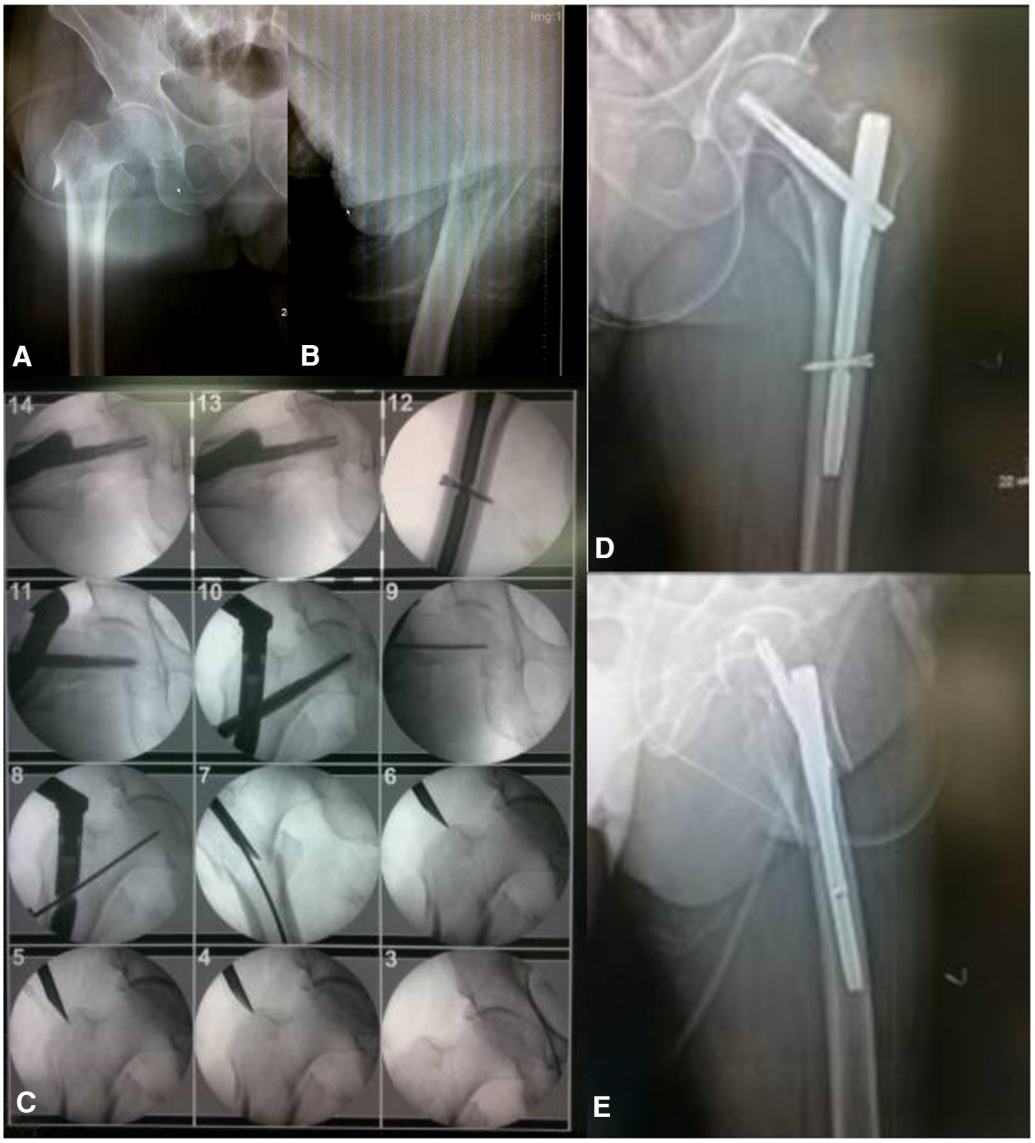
Preoperative anterolateral x-rays of the patient's hip joint (A,B) surgical procedure for PFNA (C) postoperative anterolateral x-rays of the patient's hip joint showed good fracture reduction (D,E).
